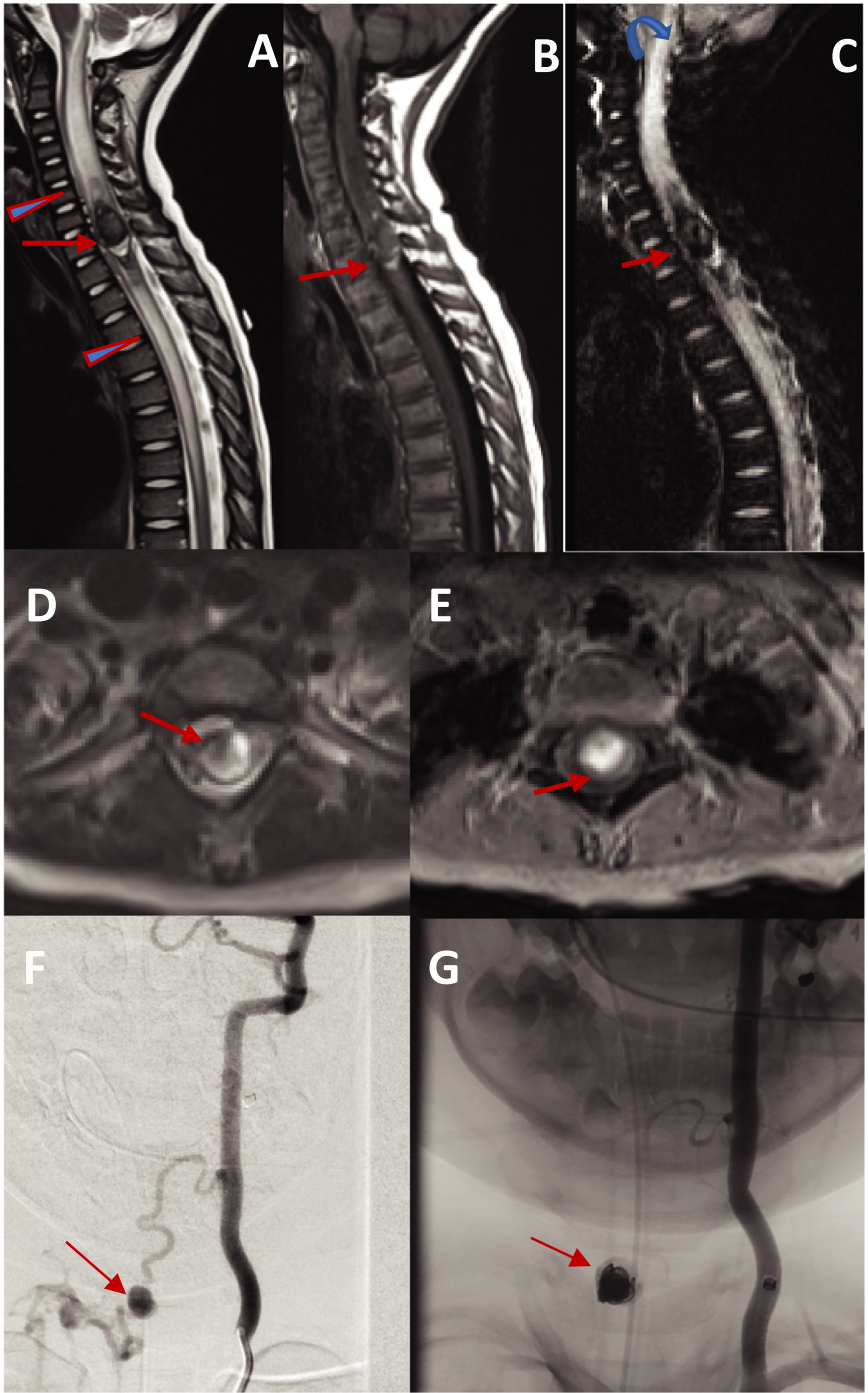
A 4‐year‐old girl presenting with acute onset quadriparesis and sensory deficit. Found to have Type IV cervical pial AVF with CM‐AVM syndrome (RASA 1 mutation): acute onset quadriparesis and sensory deficit. Sagittal T2W, T1W, and GRE MRI images of the cervical cord demonstrating long segment edema (arrow heads) in the cord, as well as a hemorrhagic lesion (arrows) at the C7/T1 level (A, B, C). Subarachnoid hemorrhage is seen as well (curved arrow) (C). Axial T2W &T1W images at C7/T1 showing products of hemorrhage (low T2, high T1, arrows) (D,E). Left vertebral artery injection demonstrating a Type IV C pial AVF with a recipient intramedullary venous varix as the site of hemorrhage (arrow). Roadmap image demonstrating microcatheter placement for embolization of the AVF (F, G).
